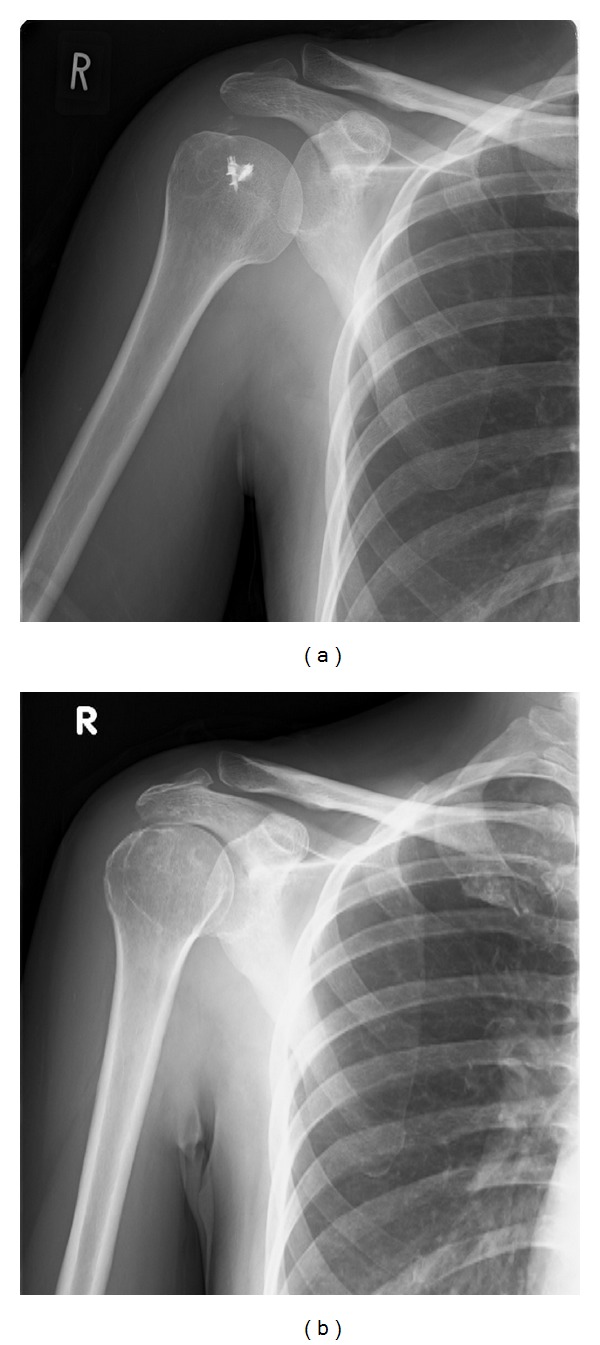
Plain radiograph (anteroposterior view). (a) Before the second surgery. (b) After the second surgery.
